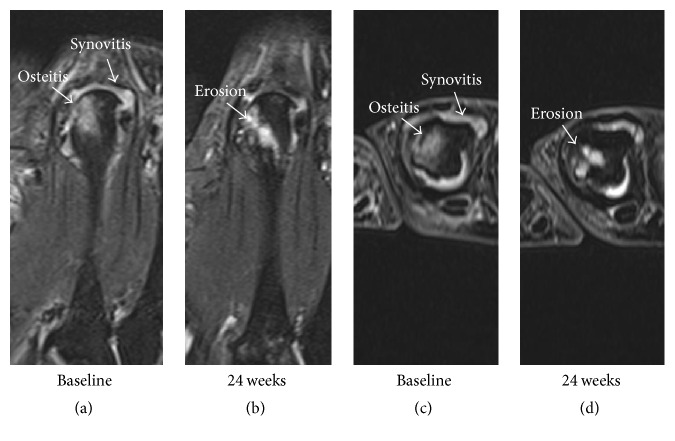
Baseline coronal (a) and axial (c) MR images of the second metacarpophalangeal joint show synovitis and osteitis but no erosion. At 24 weeks (c, d) the synovitis has decreased, and the osteitis has converted to erosion. Cartilage loss had also developed in this joint at 24 weeks (better seen on an adjacent MRI slice not shown). Without an additional time point prior to 24 weeks, however, it is not possible to determine whether the erosion developed prior to the decrease in inflammation or despite it. Further, without a time point after 24 weeks, it is not possible to determine whether the degree of residual synovitis present at 24 weeks is sufficient to drive further progression in joint damage in this case.
